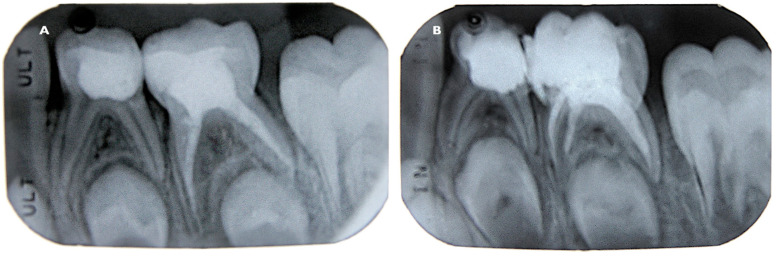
Postoperative radiographs of two mandibular second primary molars after endodontic treatment and coronal restoration (A,B).
